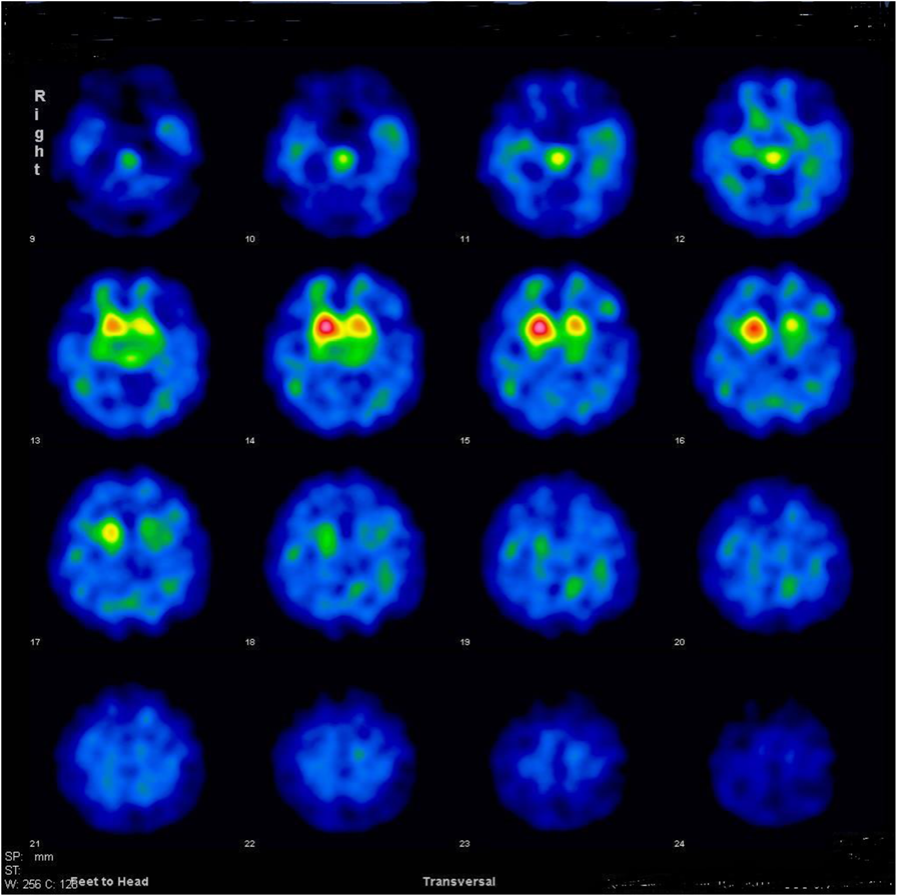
^123^I-FP-CIT (DaTscan) SPECT image results in 2015. DaTscan data demonstrate reduced bilateral striatal ioflupane (^123^I) uptake predominantly in the dorsolateral striatum. There were asymmetrical changes, with a greater decrease in ^123^I uptake in the left dorsolateral striatum
